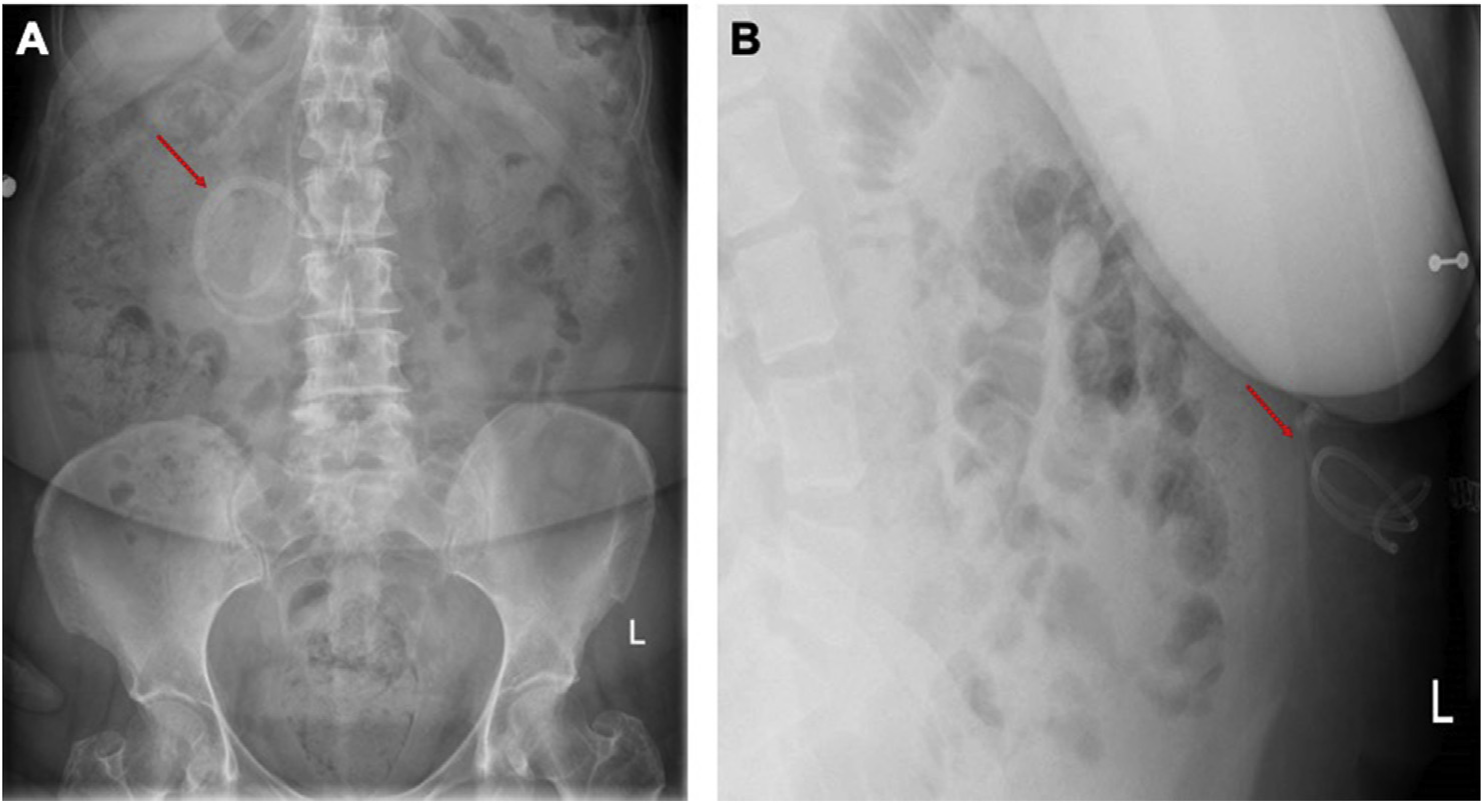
Abdominal X-rays in AP (A) and lateral (B) views in different patients showing incorrectly positioned extra-peritoneal VP shunt tubing.
